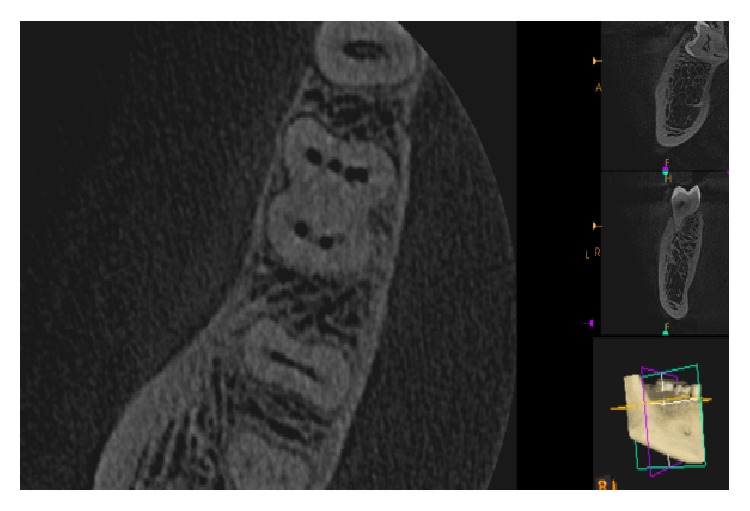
CBCT image showing 4 canals in mesial root.
